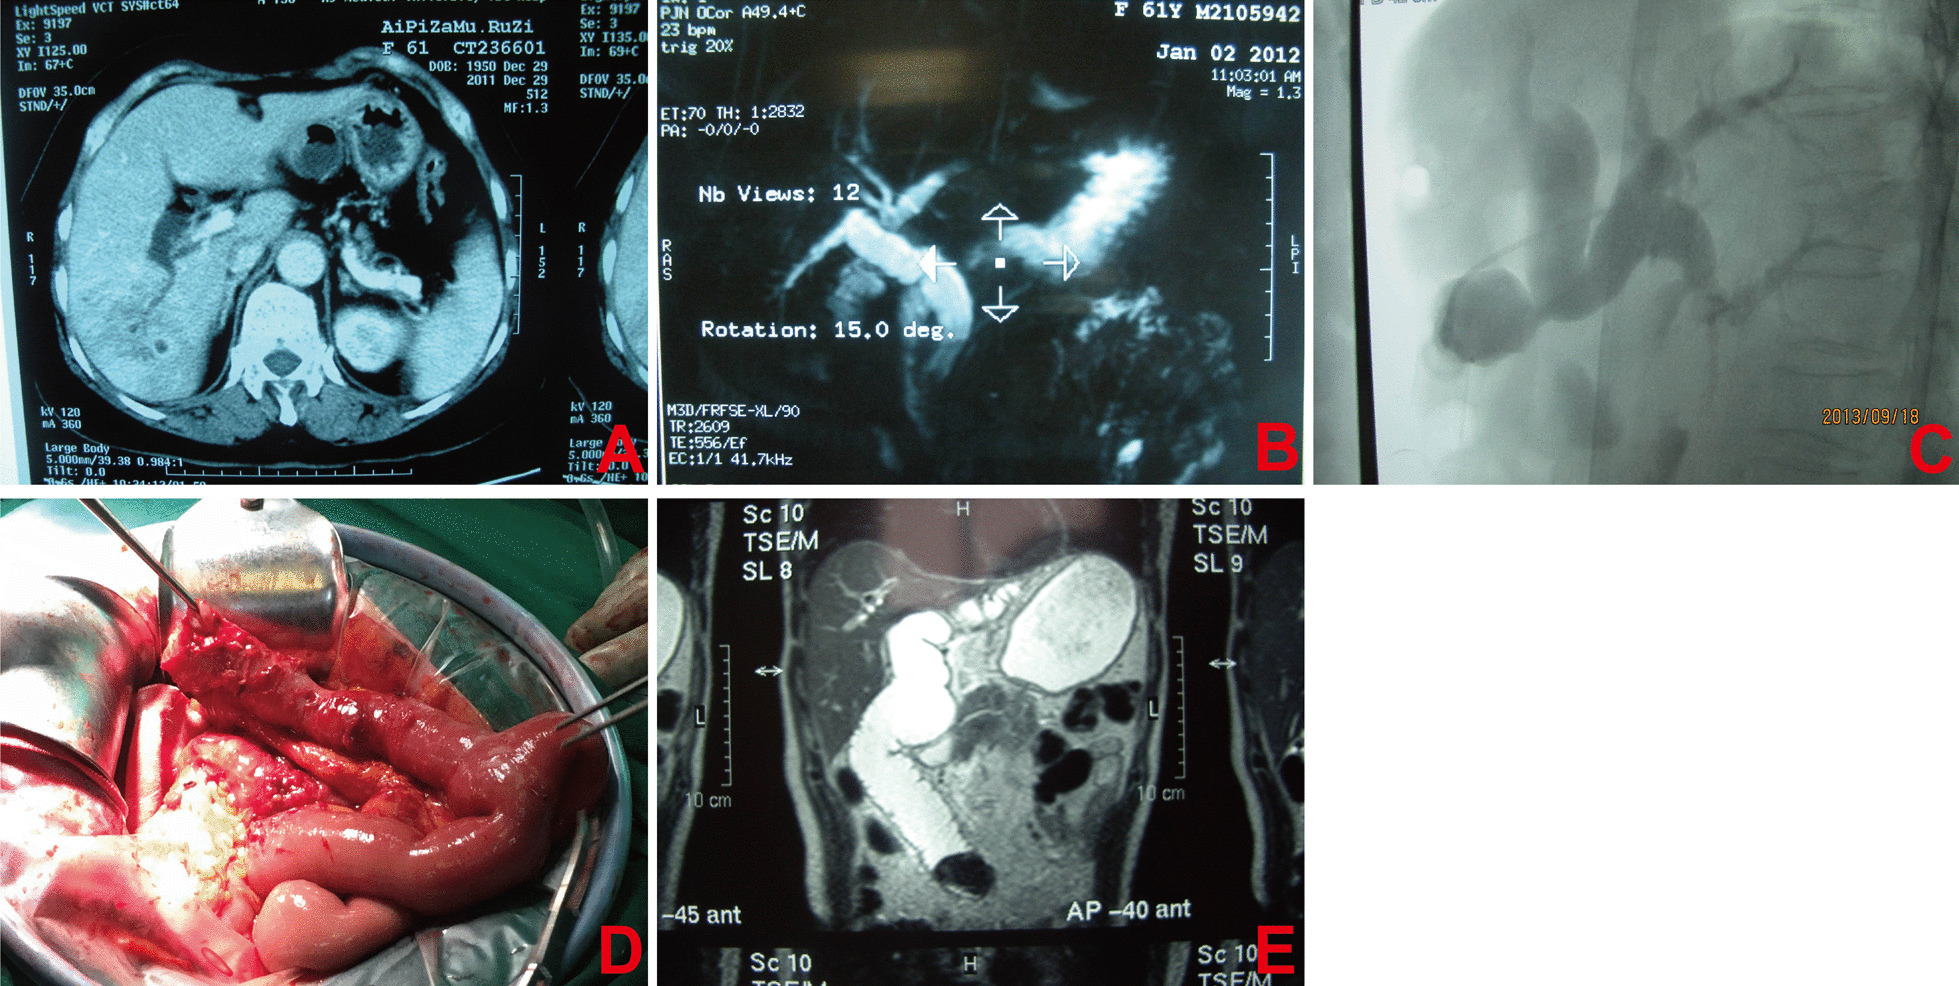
Representative imaging and intraoperative pictures of the patients with non-traumatic biliary stricture. A Enhanced computed tomography showed that the low confluence was located in the independent right posterior biliary of the common biliary duct, and there formed an abscess. B Preoperative transhepatic cholangiography indicated that the low confluence located in the independent right posterior biliary of the common biliary duct was not anatomized, and there formed calculus in the lower end of the common biliary duct. C Radiography demonstrated intestinal obstruction of biliary output loop in the near anastomotic stoma. D Intraoperative imaging showed the over-short biliary output loop. E Postoperative magnetic resonance imaging indicated calculous obstruction of biliary output loop after cholangiojejunostomy
